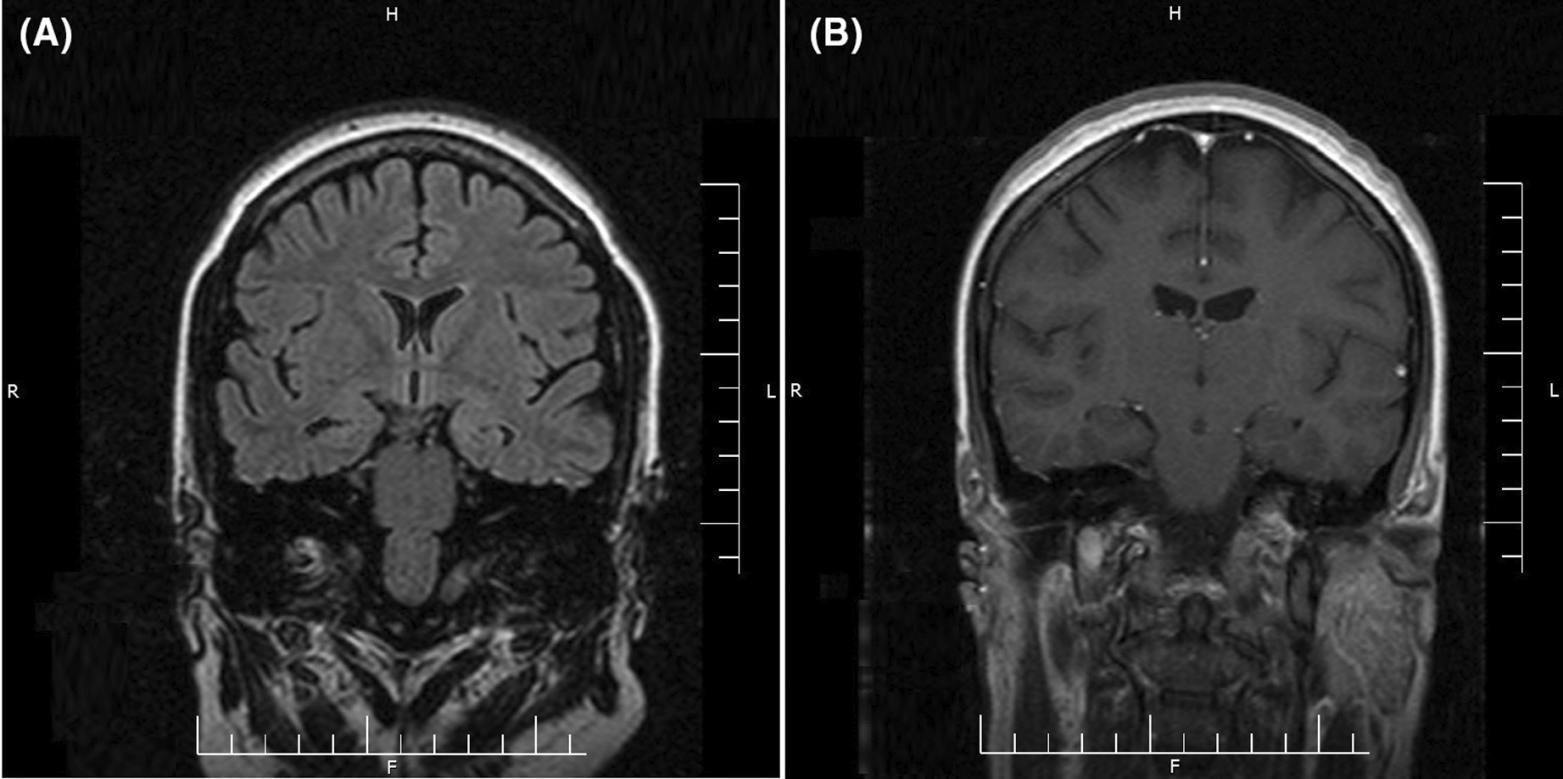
Coronal T2‐weighted Flair non‐contrast brain magnetic resonance imaging (MRI) (A) and coronal T1‐weighted contrast‐enhanced brain MRI (B)
